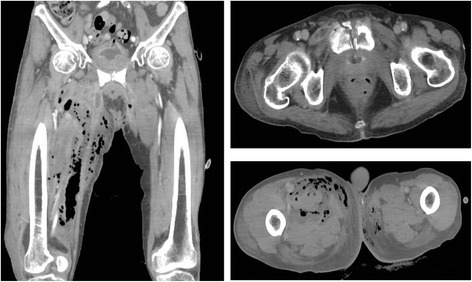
CT scan showed extensive gas within the femoral and retroperitoneal tissues and pubic bone fracture
